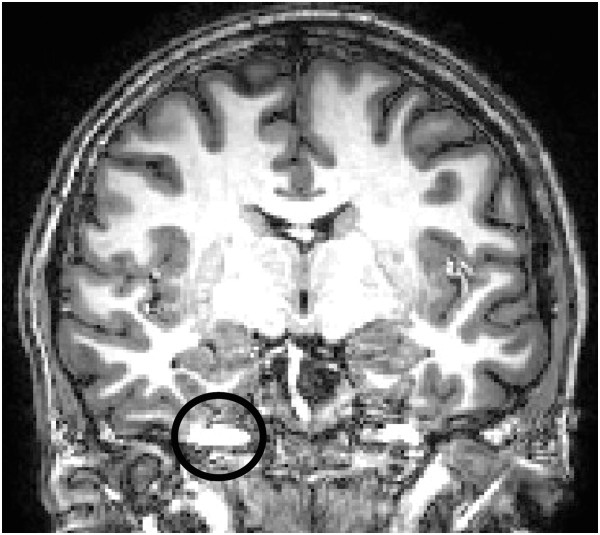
Pulsation artefact detected in the acquired images of initial T1-weighted volume protocols dataset with selected protocols C and F. Inconsistencies in phase and amplitude can result in this kind of artefact, which can be reduced by adding flow compensation and/or changing the phase encoding direction.
